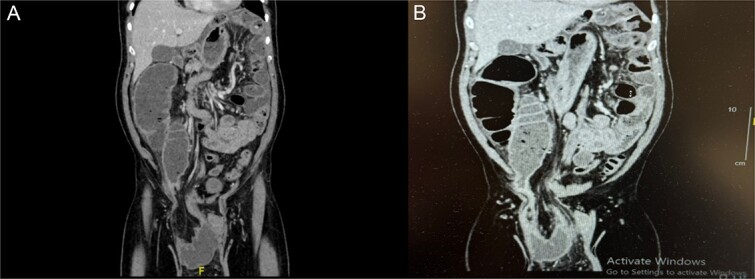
(A, B) Coronal abdominal CT showing right side inguino-scrotal hernia that contains mesenteric fat and vessels with long segment of transverse colonic loop, there is proximal dilatation of the right side of the colon reaching a competent ileo-cecal valve and not associated with dilatation of the small bowel loops. The left side colon is collapsed.
